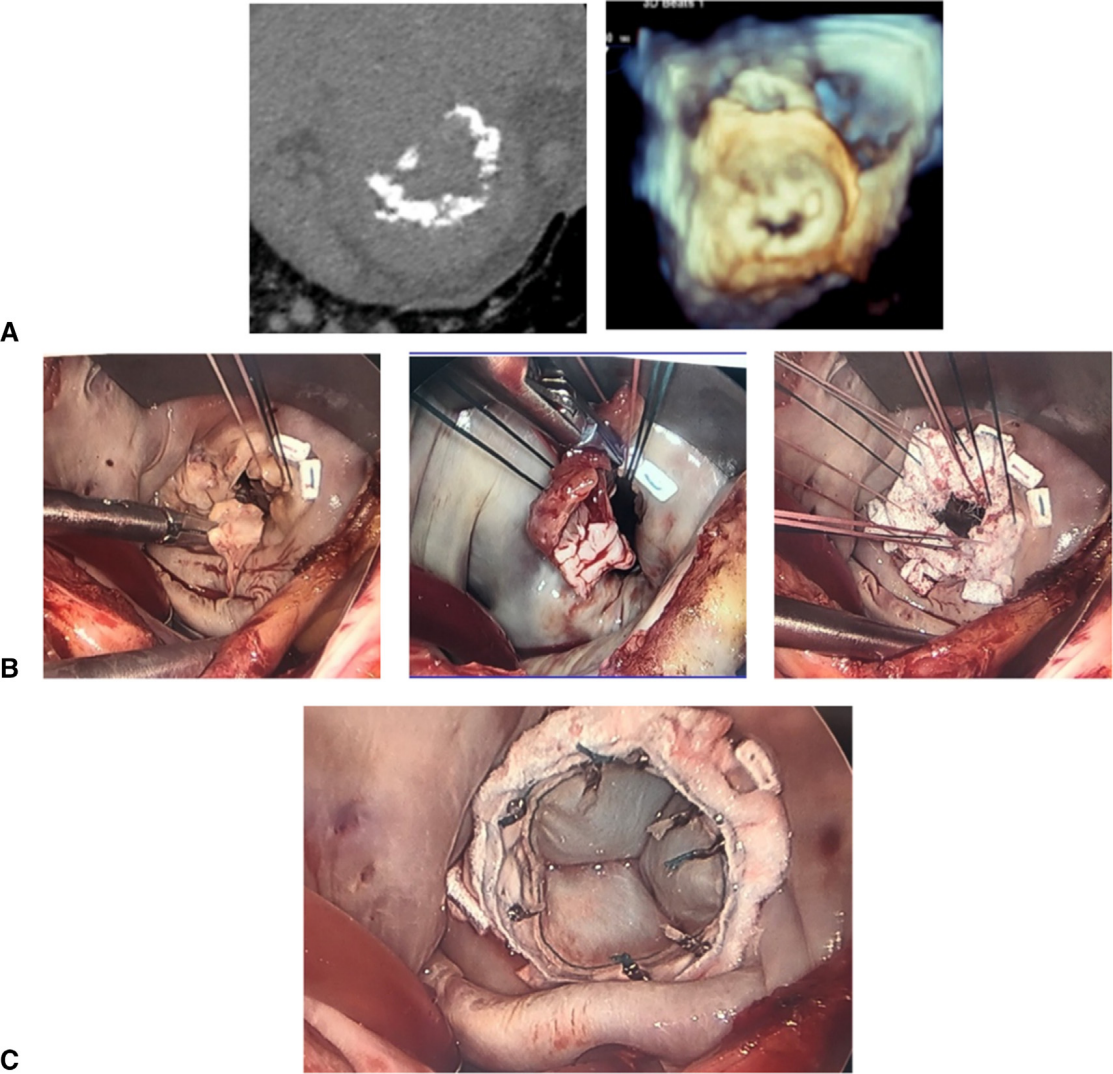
Surgical implantation of a transcatheter valve. A, Preoperative CT and 3D echocardiogram demonstrating severe MAC. B, Intraoperative images demonstrating removal of A2 segment (*right panel*), septal resection (*middle panel*), and placement of felt strip (*left panel*). C, Final implantation of Sapien 3 valve.
